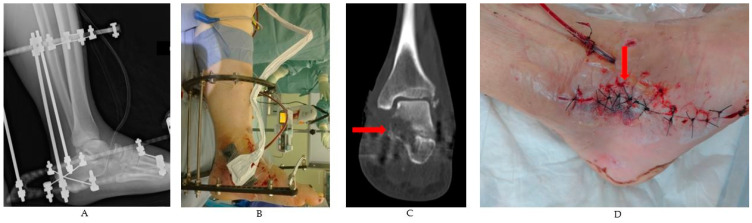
Early postoperative course during continuous local antibiotic perfusion and temporary external fixation. (A) Lateral radiograph obtained after the initial surgery demonstrating stabilization of the talonavicular and subtalar joints with external fixation. (B) Clinical appearance during CLAP therapy showing persistent but improving local inflammation of the medial ankle. (C) Computed tomography images demonstrating postoperative changes following curettage of infected bone in the talonavicular and subtalar joints (red arrow). (D) Clinical appearance at postoperative day 14 showing marked improvement of local inflammatory signs following debridement and CLAP therapy (red arrow).
